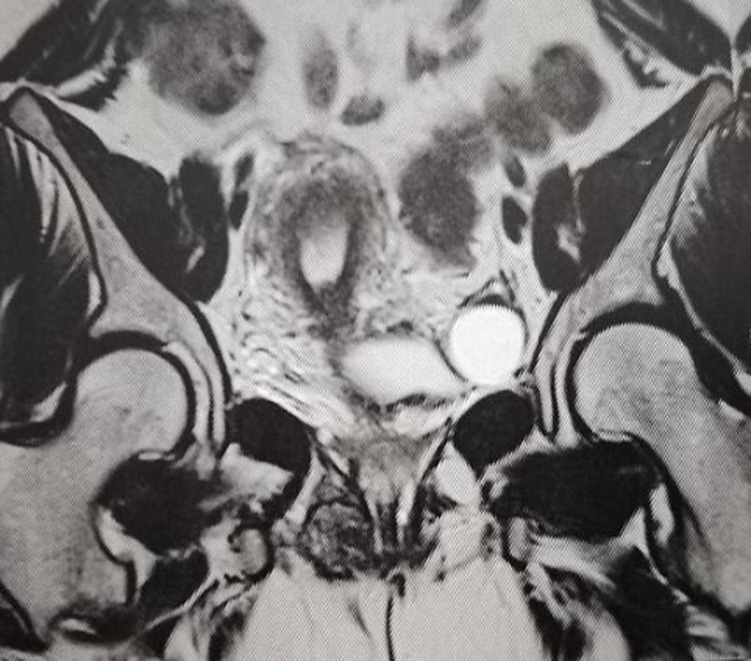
T2-weighted MRI image, coronal plane through pelvis showing the endometriotic nodule (arrow)
